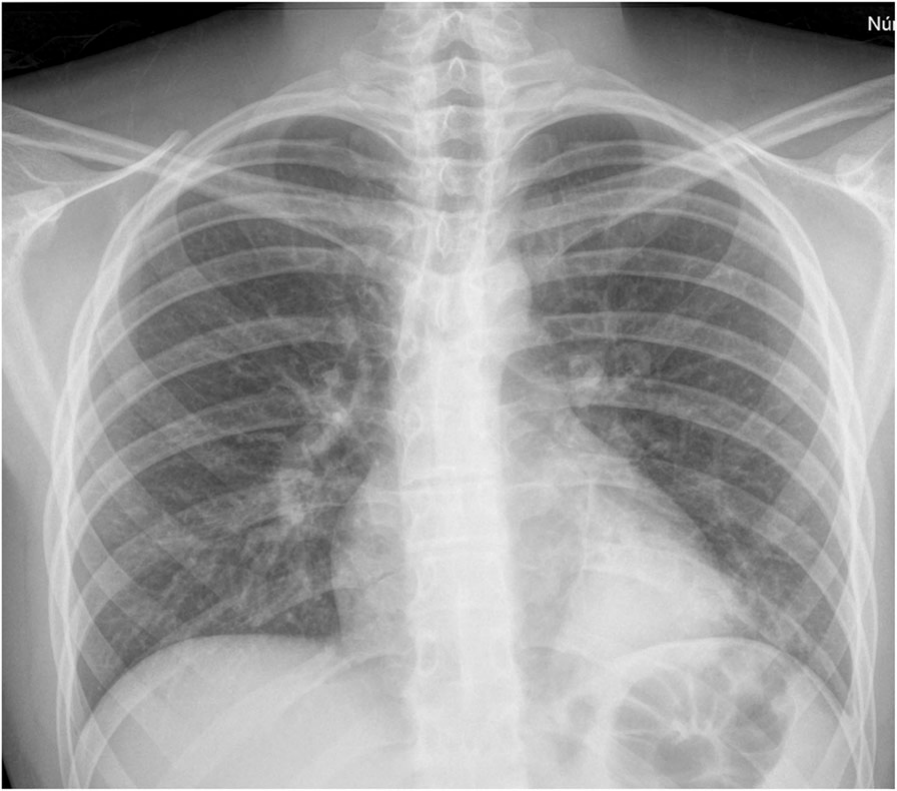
Chest x-ray performed on his first emergency department visit
